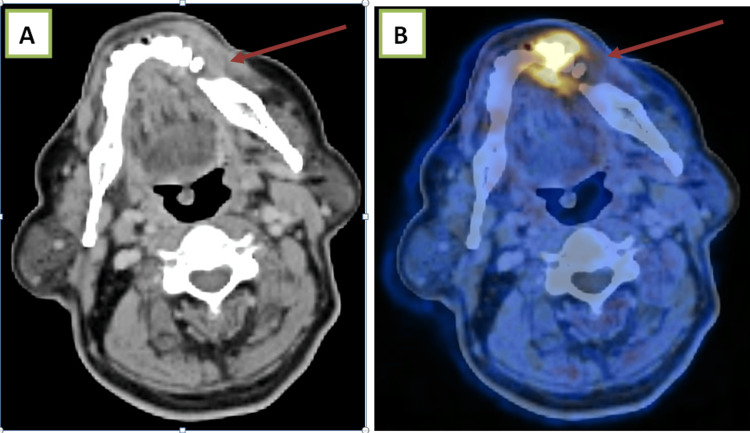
(A) Contrast-enhanced CT and (B) fused PET-CT images show recurrent soft tissue thickening and adjacent osseous erosion. PET-CT, positron emission tomography-computed tomography
